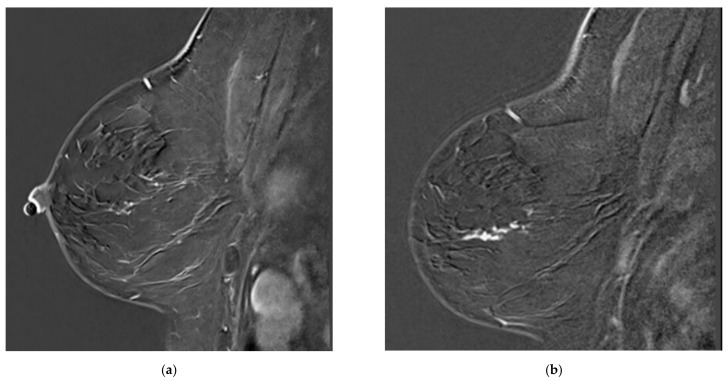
Examples of missed lesions. Overlooked linear non-mass enhancement: (a) MR A; (b) follow-up MRI (MR B) one year later showing more conspicuous non-mass enhancement, corresponding to DCIS.
